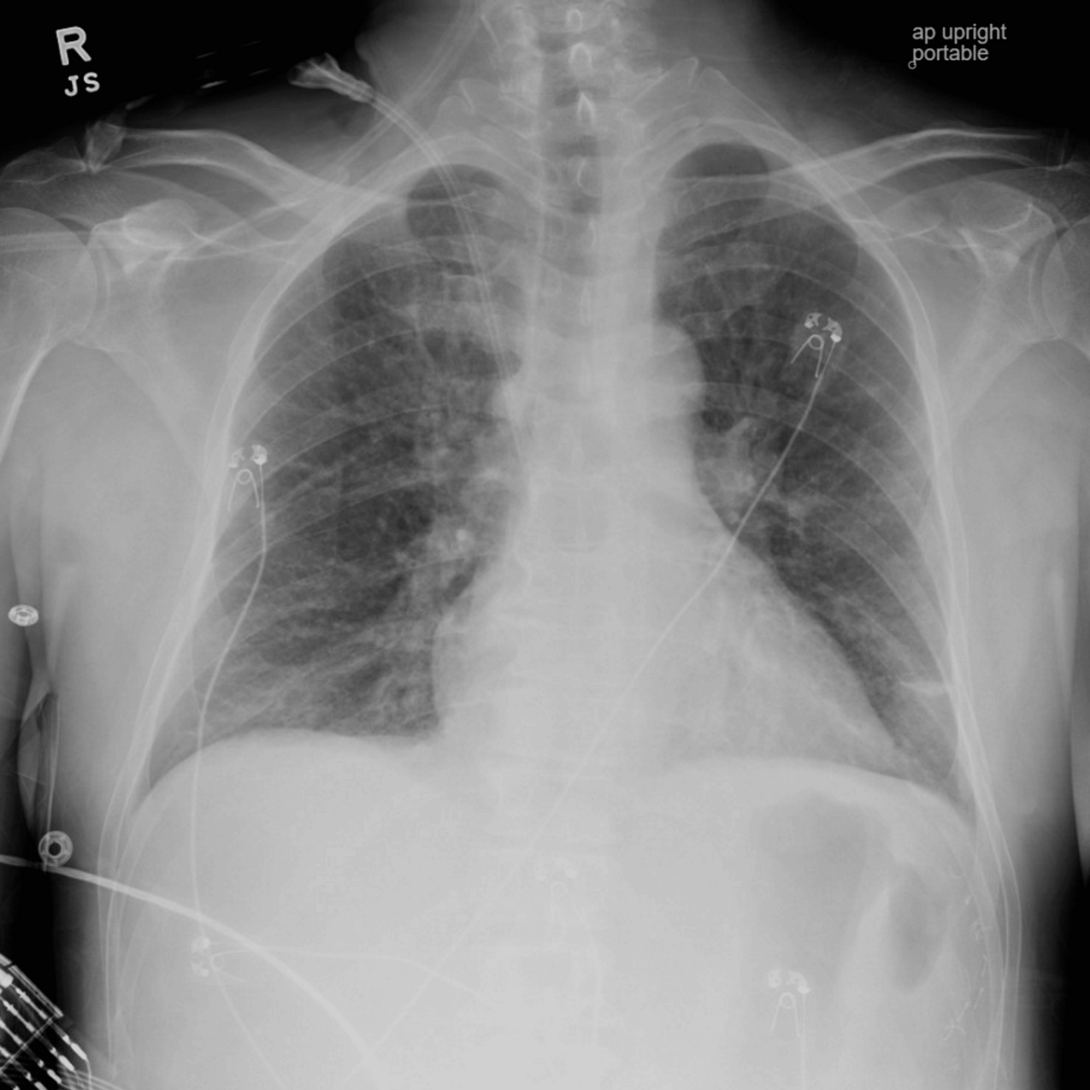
Chest X-ray at admission showing bilateral lower lobe atelectasis
